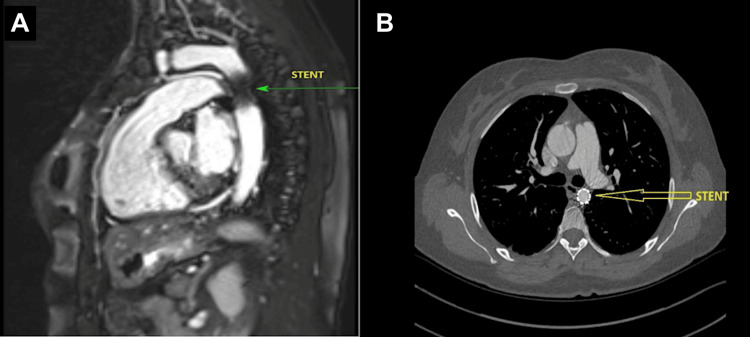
Post-procedure follow-up MRI (A) and CT aortogram (B) showing stent in situ
